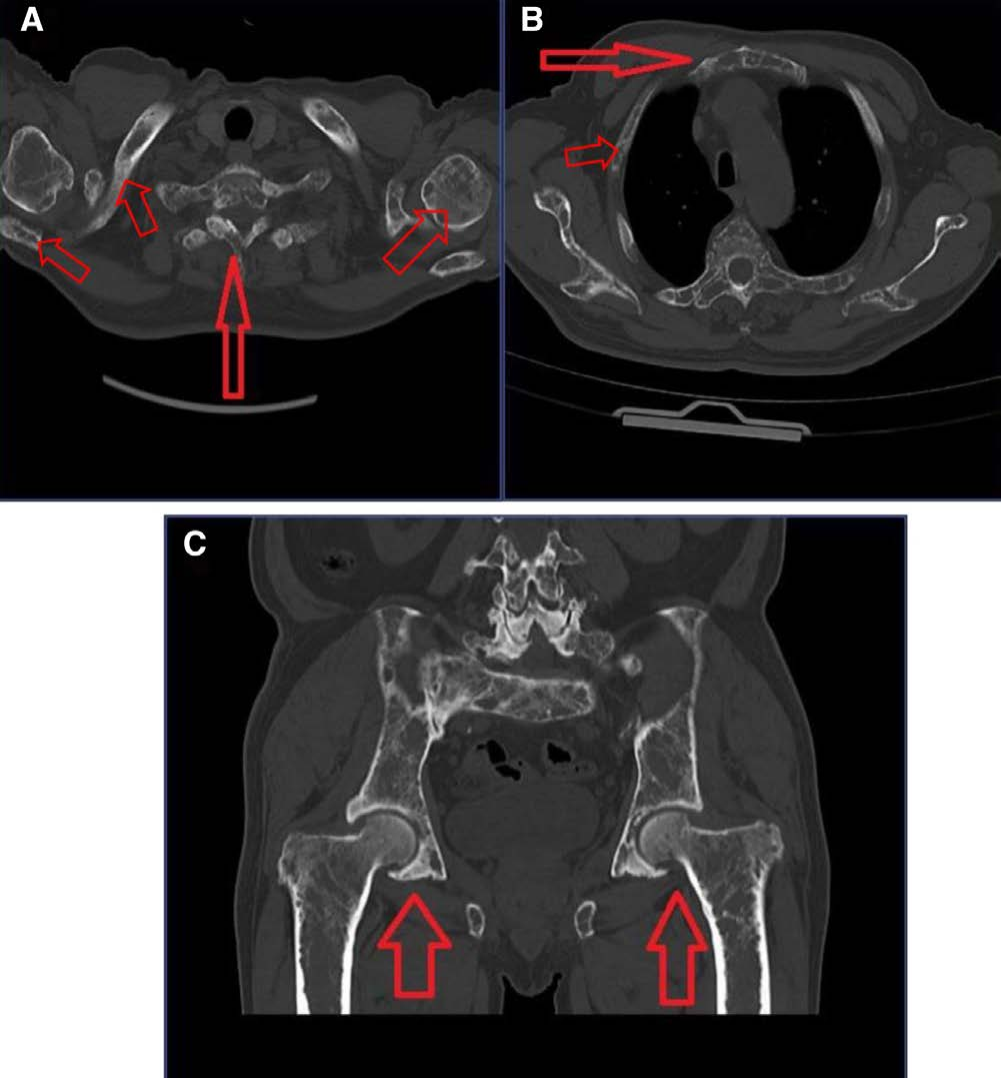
Low-dose whole-body bone CT: multiple bone destruction of the sternum, ribs, multiple vertebrae and accessories of the spine, pelvis, bilateral humerus and proximal femur. CT = computed tomography.
